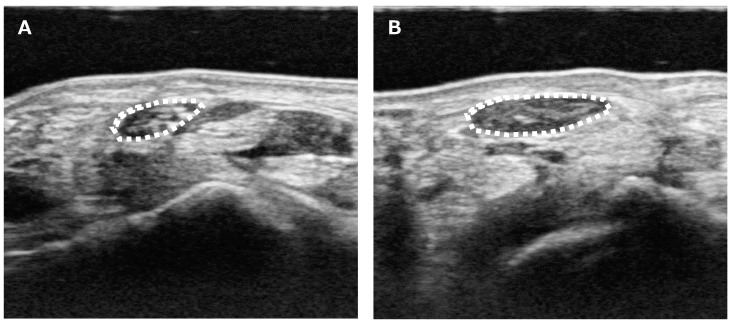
Ultrasound measurement of the median nerve CSA. (A) Unaffected side. (B) Affected side. CSA was measured as the only parameter for the image analysis. Median nerve CSA was measured at the wrist crease level. The median nerve was outlined (white dotted line), and its area was calculated.
